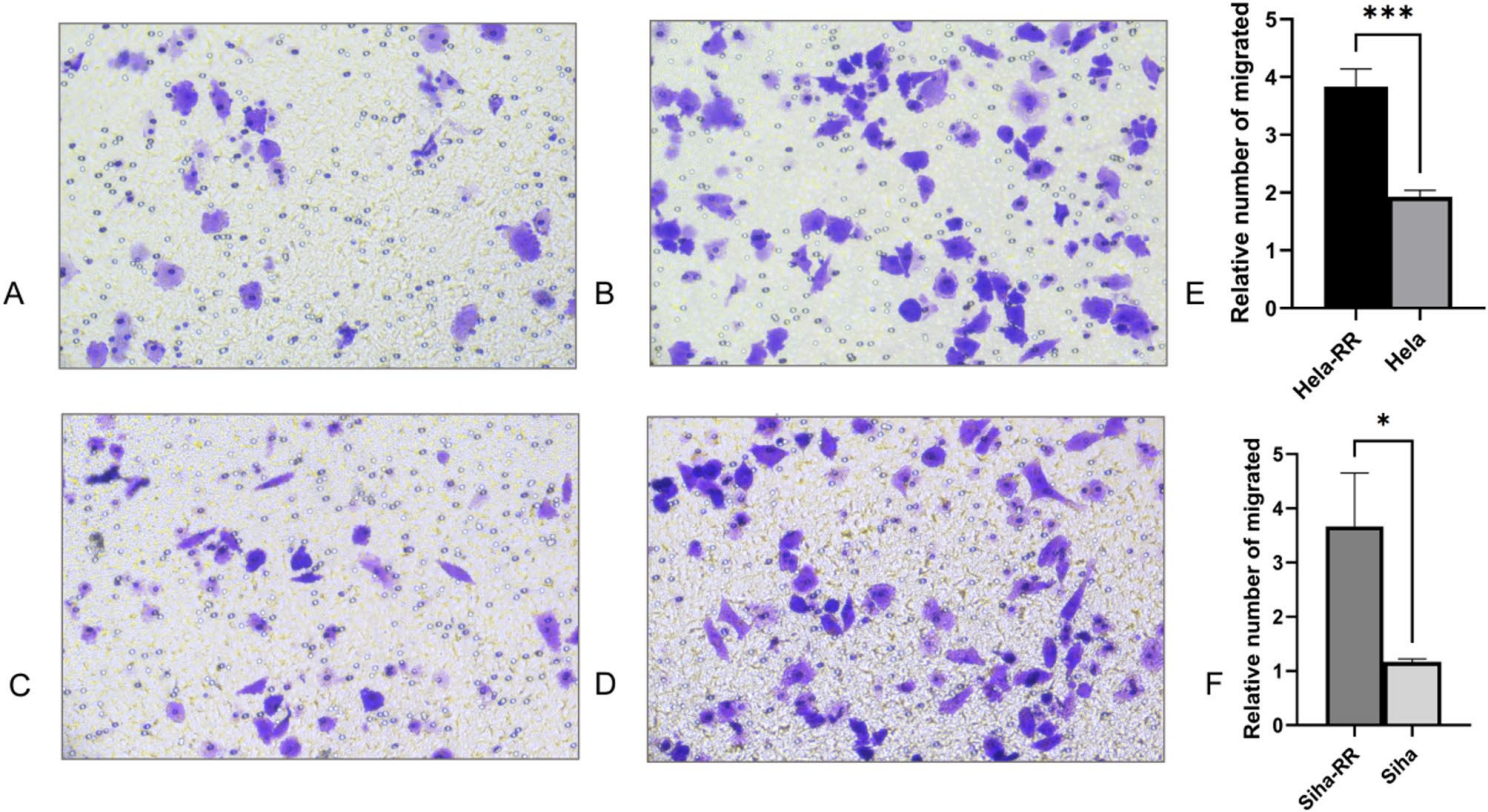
Acquired radiotherapy-resistant cell lines are more invasive after radiation exposure. A Representative images of cell invasion capacity assay of HeLa cells after radiation with a single 6 Gy dose of 6 MeV X-rays. B Representative images of cell invasion capacity assay of HeLa-RR cells after radiation with a single 6 Gy dose of 6 MeV X-rays. C Representative images of cell invasion capacity assay of SiHa cells after radiation with a single 4 Gy dose of 6 MeV X-rays. D Representative images of cell invasion capacity assay of SiHa-RR cells after radiation with a single 4 Gy dose of 6 MeV X-rays. E Quantitative comparison of capacity to invasive after radiation exposure between HeLa-RR and HeLa cells. F Quantitative comparison of capacity to invasive after radiation exposure between SiHa-RR and SiHa cells. All data are presented as mean ± standard deviation, Statistical significance was determined by two-tailed Student’s t-test. (Significance markers: P* < 0.05, *P* < 0.01, P* < 0.001; ns = not significant (*P* > 0.05).)
